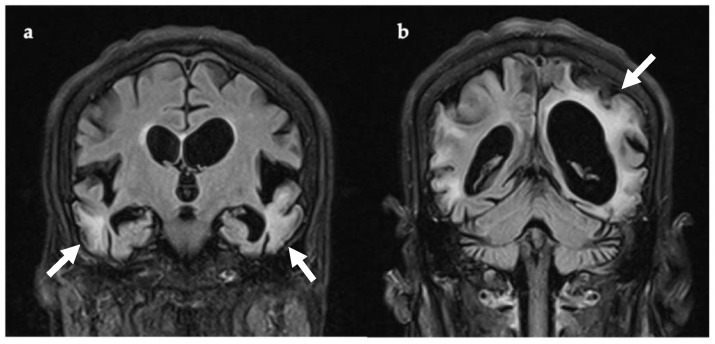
Coronal T2-FLAIR MRI, 21 days after symptom onset. There are multiple areas of supra-tentorial encephalomalacia bilaterally within the temporo-parieto-occipital lobes with parenchymal T2 signal change, thinning of gyri, and white matter volume loss ((a,b), white arrows). Multiple vascular territories are involved. Abbreviations: FLAIR, fluid attenuated inversion recovery imaging.
